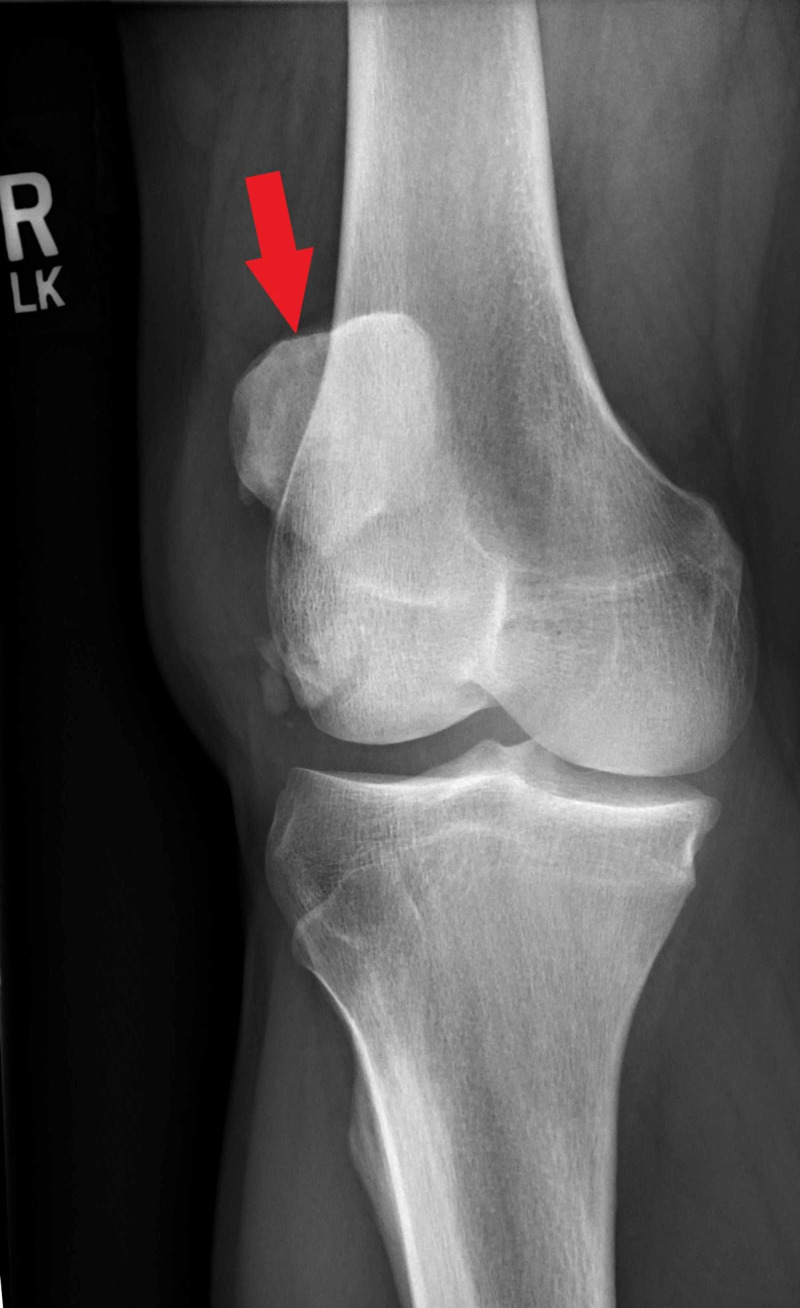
Anterior-posterior view radiograph of the right knee demonstrating high-riding patella (red arrow)
